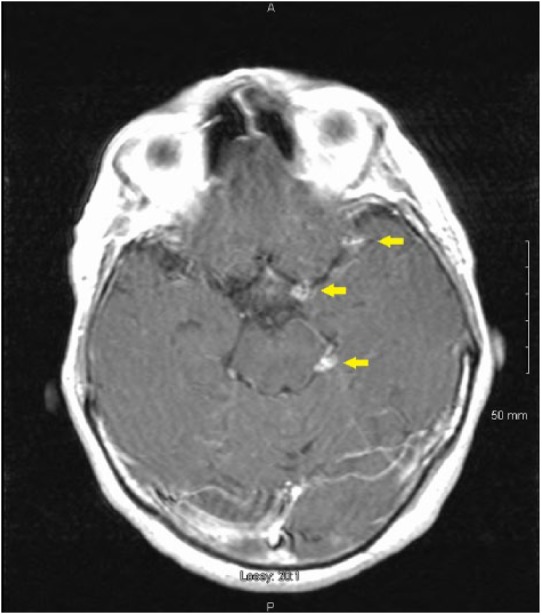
T1-weighted magnetic resonance image with gadopentetate dimeglumine contrast showing several nodular enhancements in the left peripontine area suspicious for coccidioma (arrows) in patient with multiple consecutive crescendo cerebrovascular transient ischemic attacks.
